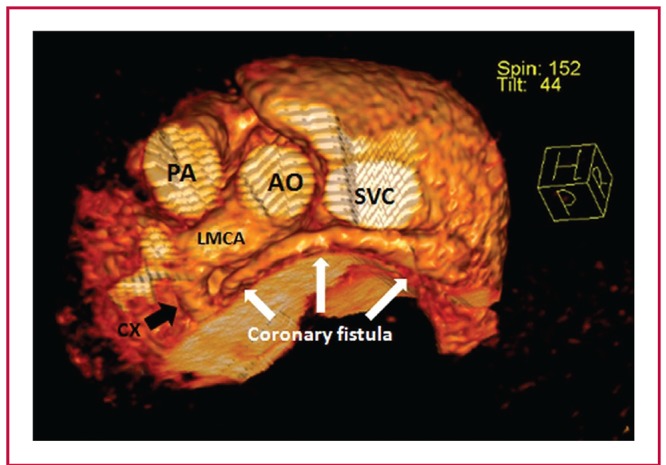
Three-dimensional reconstructed computed tomographic view demonstrating the trajectory of the coronary fistula. Note the dilated left main and circumflex coronary arteries. AO: aorta, LMCA: left main coronary artery, PA: pulmonary artery, SVC: superior vena cava.
